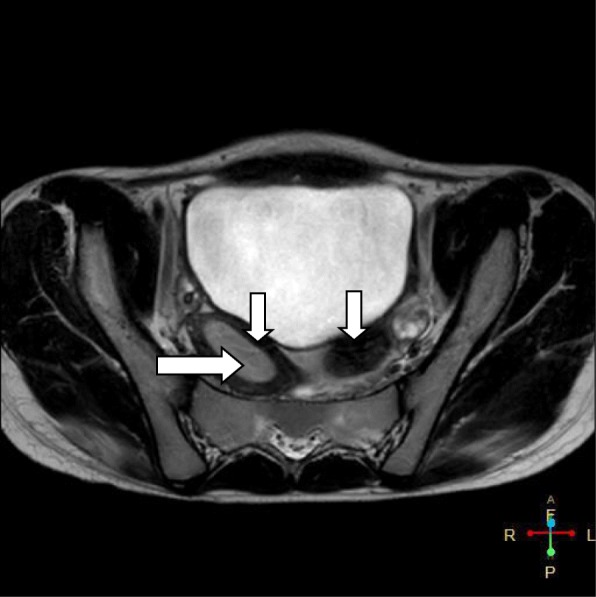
Axial T2-weighted image showing two separate uterine horns (*short arrows*) and distention of right endometrial cavity (*long arrow*)
